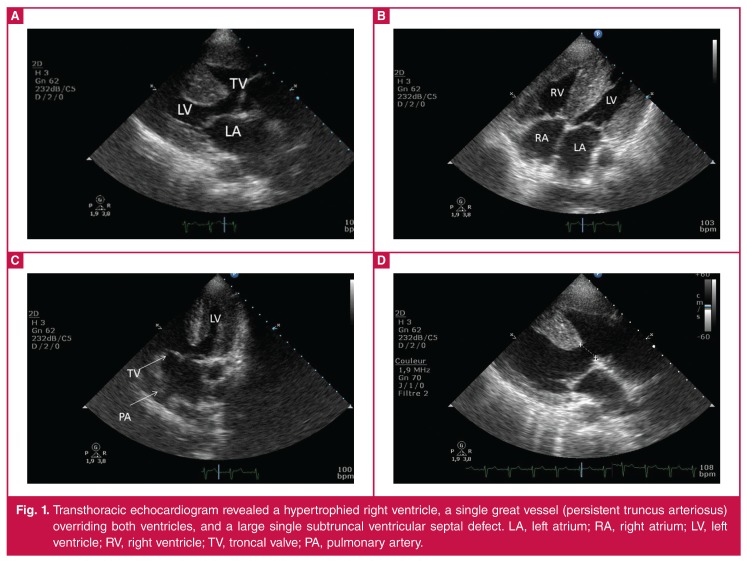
Transthoracic echocardiogram revealed a hypertrophied right ventricle, a single great vessel (persistent truncus arteriosus) overriding both ventricles, and a large single subtruncal ventricular septal defect. LA, left atrium; RA, right atrium; LV, left ventricle; RV, right ventricle; TV, troncal valve; PA, pulmonary artery.
